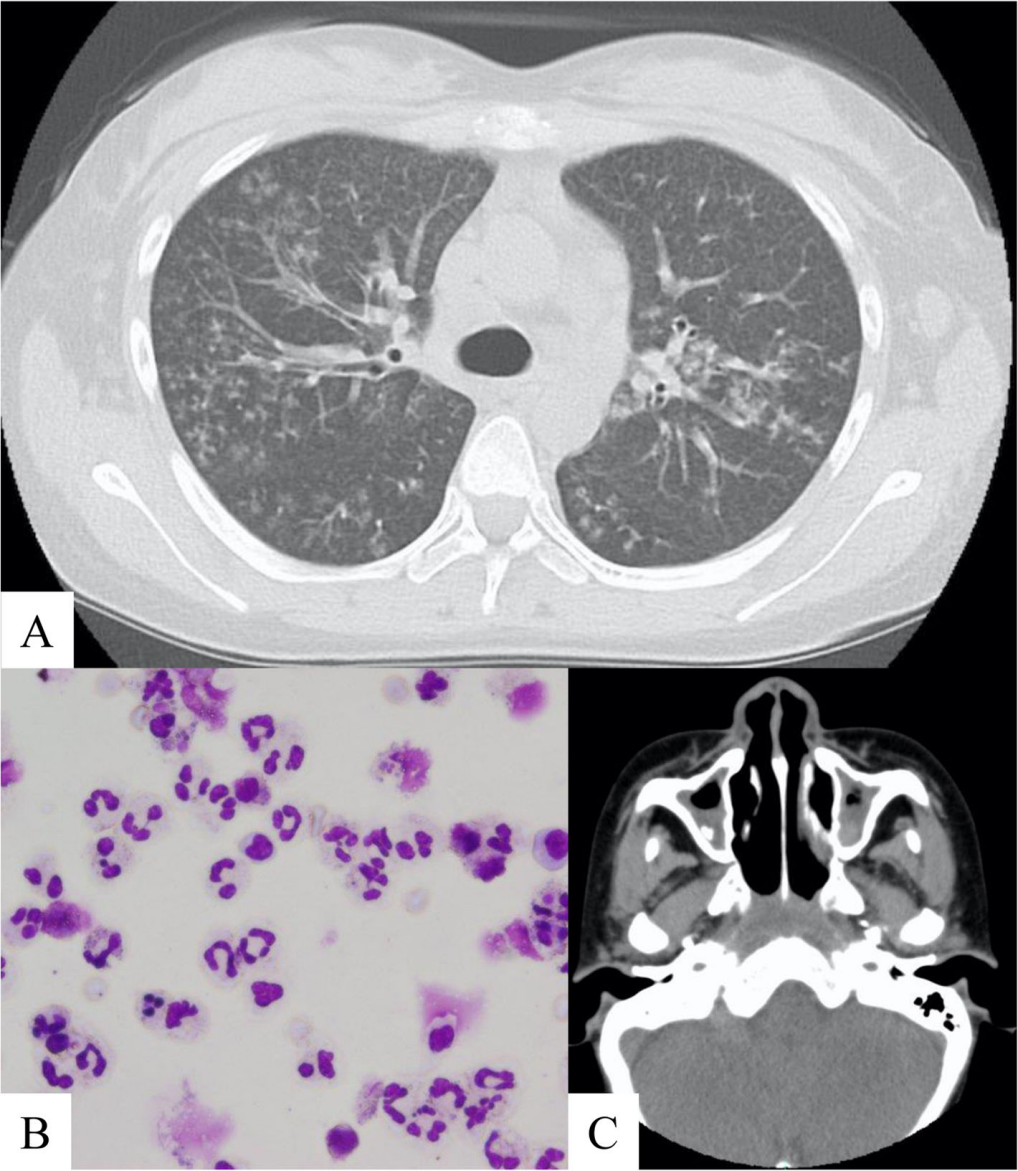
a Chest computed tomography (CT) scan revealed bronchial wall thickening and presence of centrilobular nodules 3 months after splenectomy. b The second analysis of the bronchoalveolar lavage fluid revealed a remarkable increase in neutrophil count. c Paranasal sinus CT scan revealed thickening of the maxillary sinus wall
